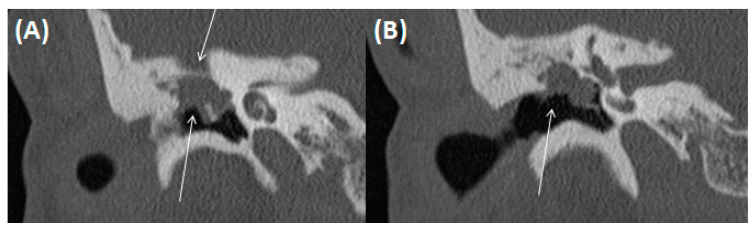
A 51-year-old male patient with cholesteatoma. Coronal CT using bone windowing. A hypodense mass is present in the epitympanum (arrows) with bony destruction of the scutum and beginning destruction of the ossicular chain. Note the beginning invasion towards the intracranial compartment. Figure (A,B) shows the middle ear in coronal view at different levels.
